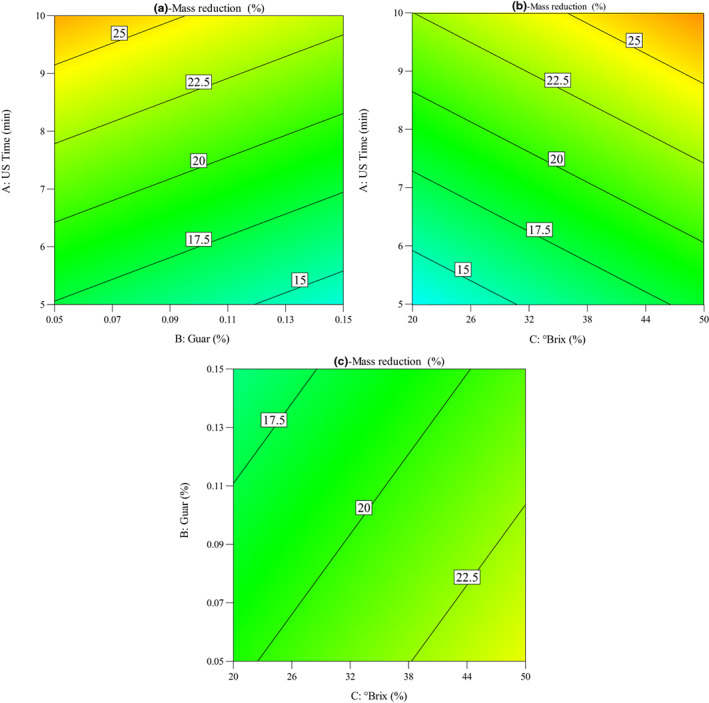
Contour plots for the impacts of operating conditions on the mass reduction of quince slices: (a) ultrasound time versus guar concentration, (b) ultrasound time versus °Brix, and (c) guar concentration versus °Brix.
